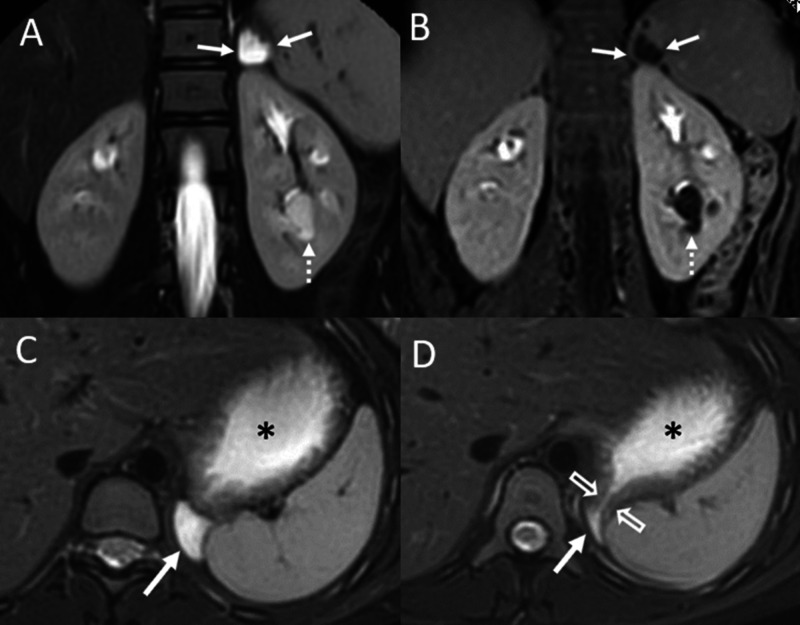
Gastric diverticulum diagnosed on an MR urogram. Coronal (A) T2-weighted and (B) T1-weighted contrast enhanced MR images show a left supra-renal lesion (solid arrows) that is increased signal intensity on the T2-weighted sequence (A) and shows peripheral enhancement on the post-contrast image (B). Consecutive axial T2-weighted images (C and D) show the close proximity of the lesion (solid arrows) to the stomach (asterisk) with the more superior image (D) showing a communication (open arrows) with the stomach. The dashed arrows (A and B) show a peri-pelvic left renal cyst.
